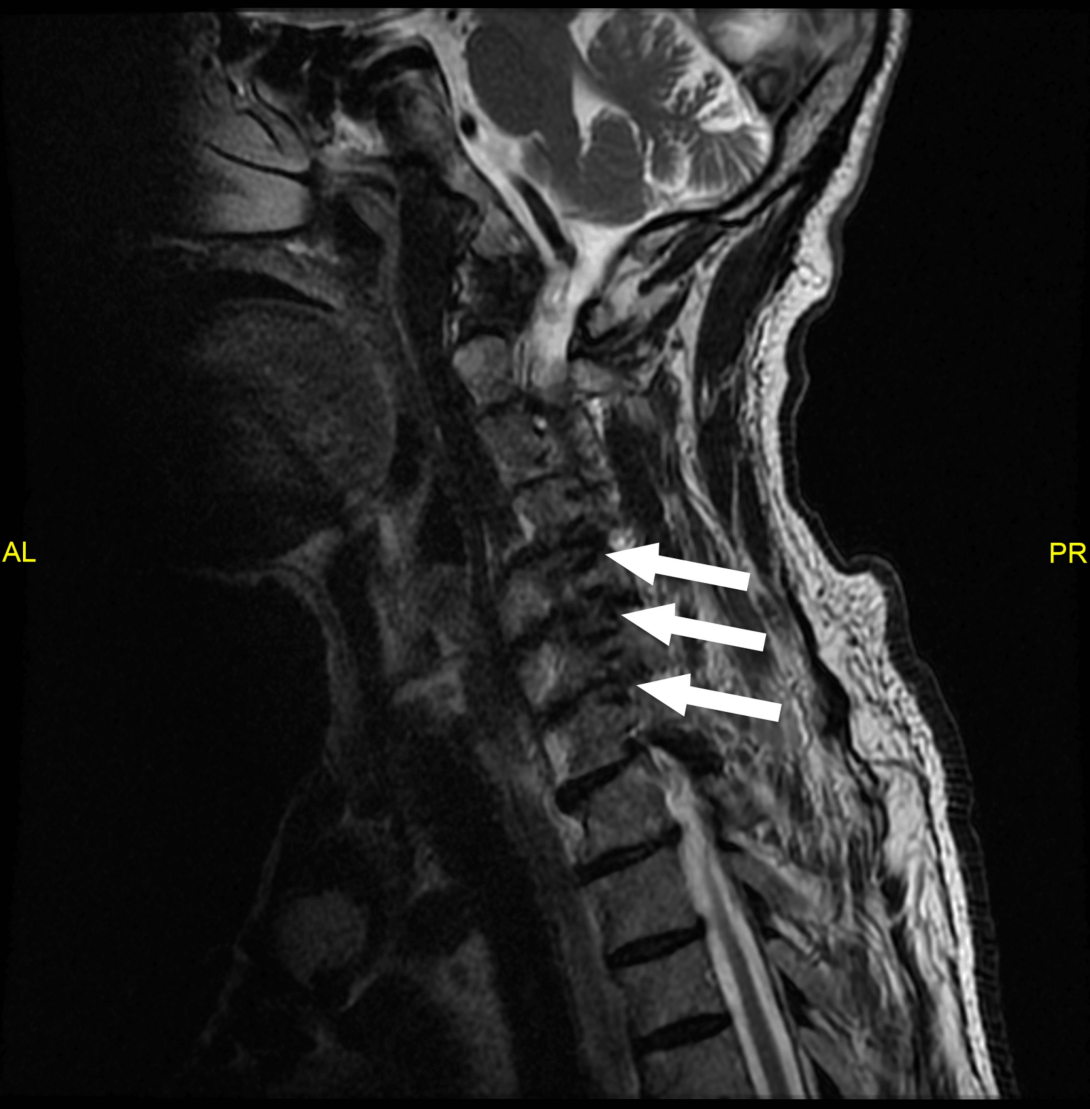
Sagittal T2W image through the cervical spine showing the stenosed C4-C7 neuroforamina (arrows) T2W: T2-weighted
